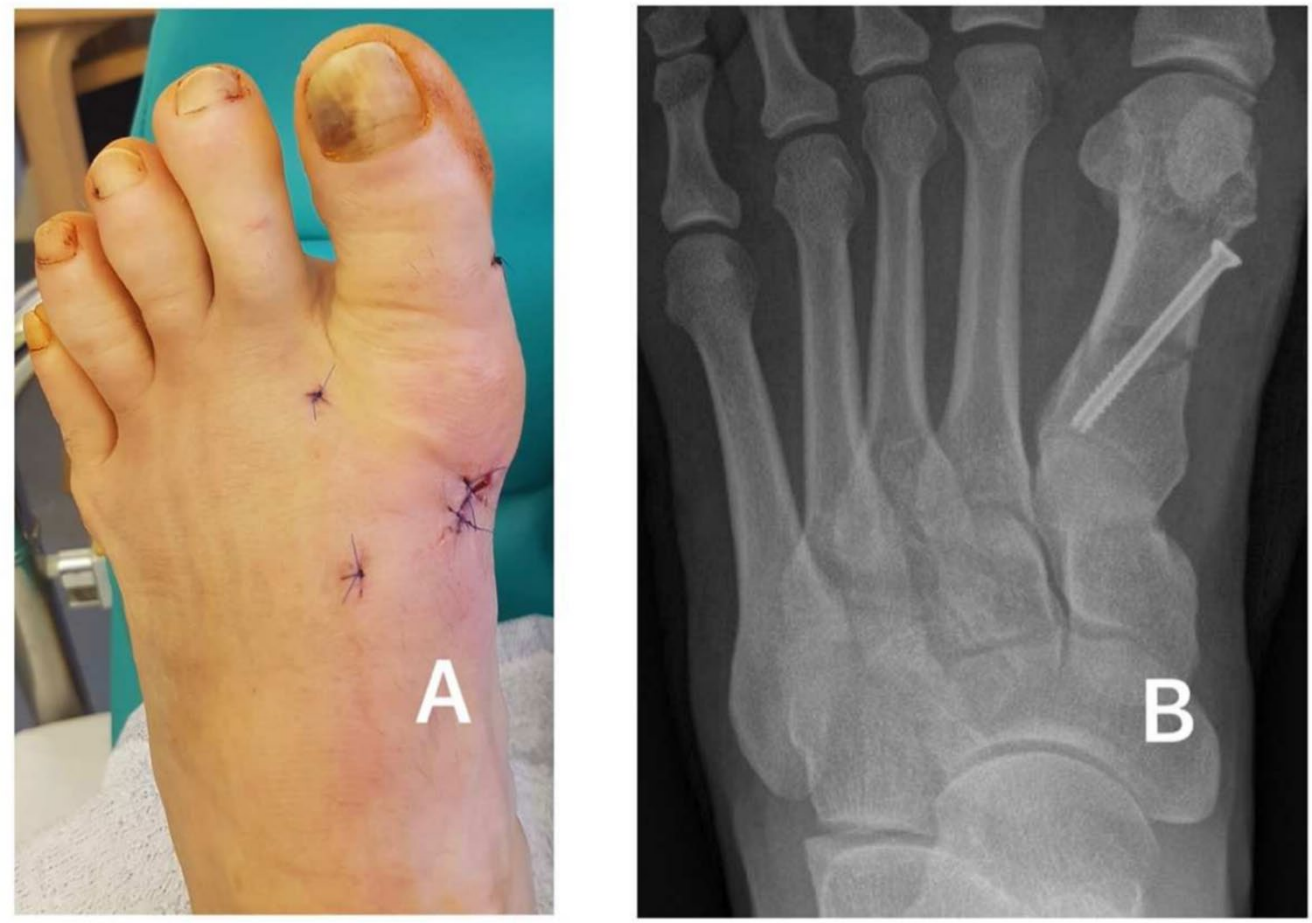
A: Post-operative clinical image, A and X-ray, B after minimally invasive proximal osteotomy and screw fixation
